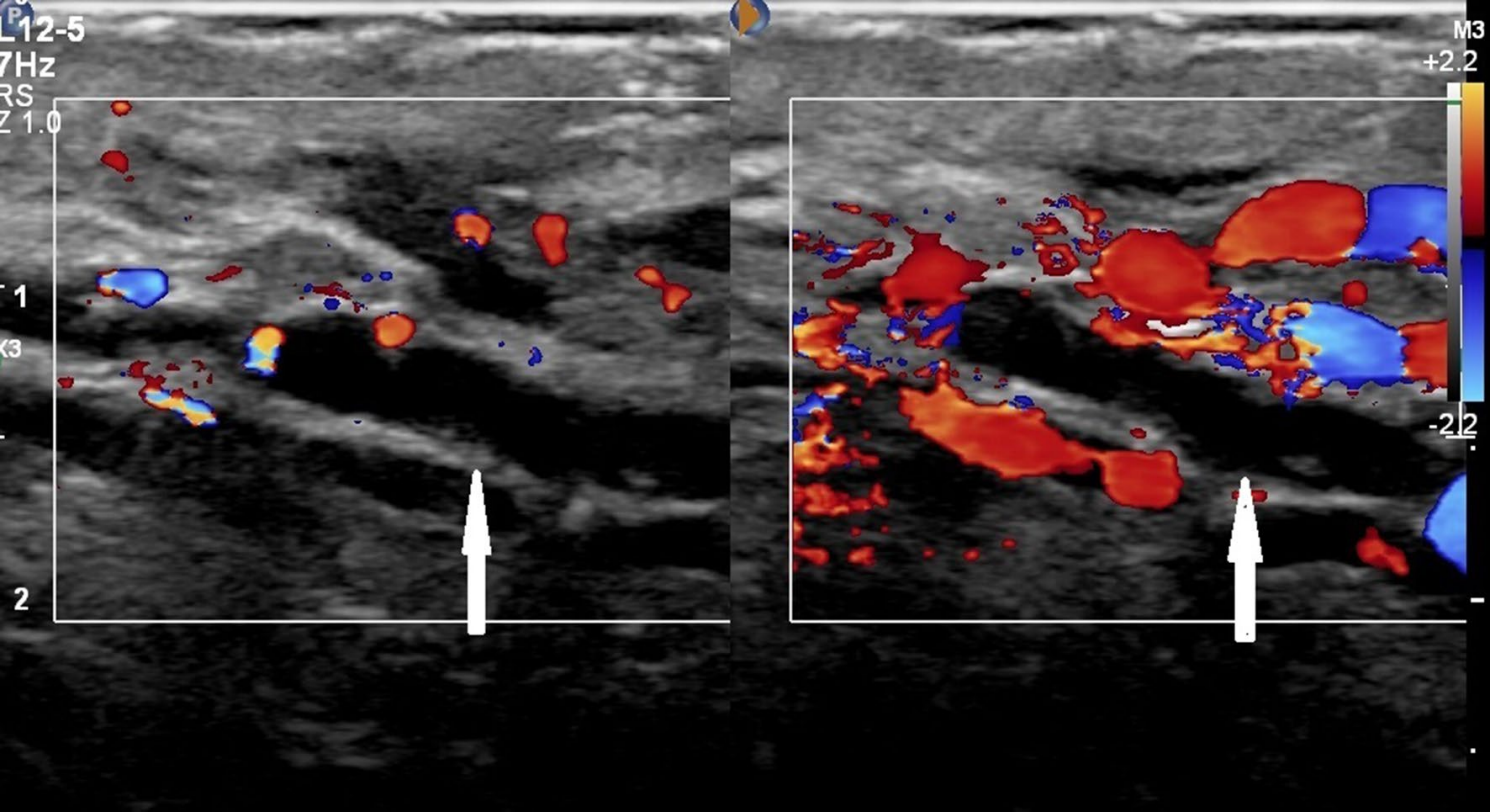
A 28-year-old man with dilated peritesticular veins. The veins of left pampiniform plexus present fluid-filled anechoic tubular structures in the region of the spermatic cord on duplex ultrasound at supine position without Valsalva maneuver (arrow, left panel); some of the fluid-filled anechoic tubular structures show color Doppler flow signal on duplex ultrasound at standing position with Valsalva maneuver, and the others do not show color Doppler flow signal (right panel). The scrotum does not show edematous and thicken.
